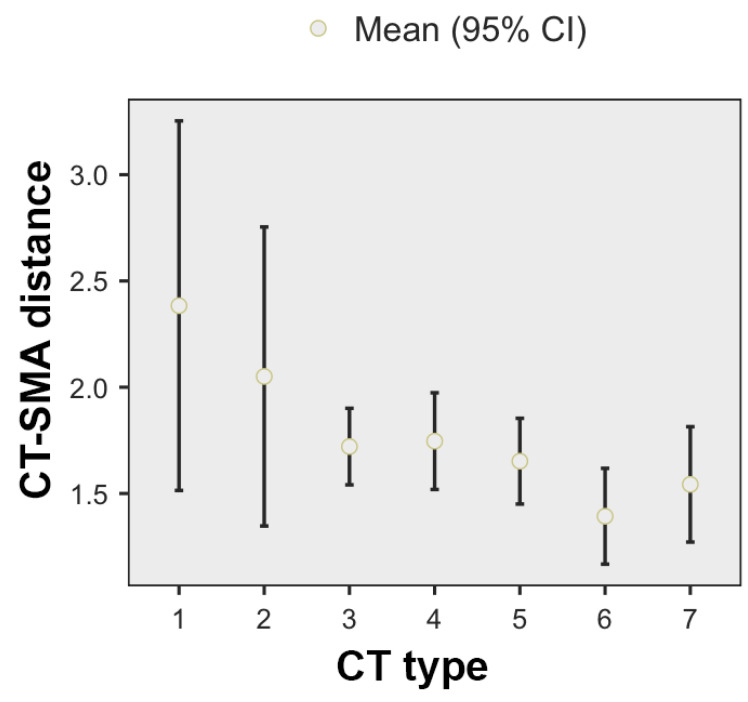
CT–SMA distance, depending on the CT type.
